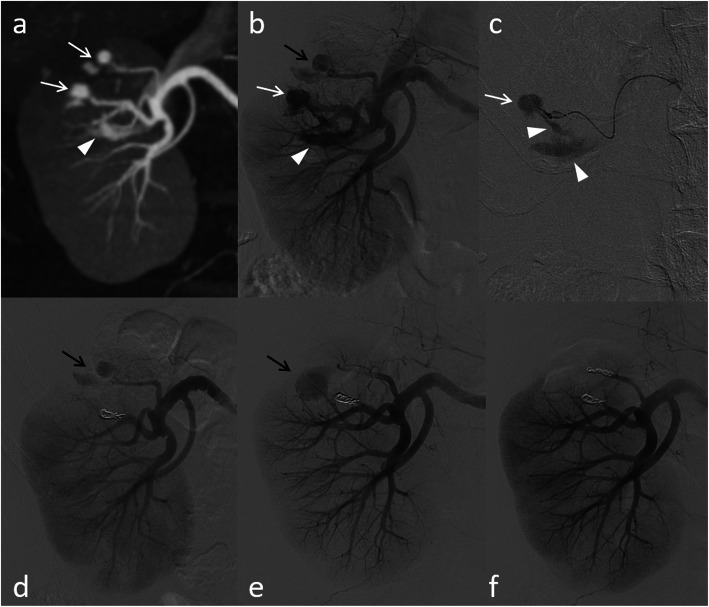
A case of undergoing transarterial embolization (TAE) twice in a 75-year-old man. a Two renal artery pseudoaneurysms (RAPs) (arrows) with arteriovenous fistula (AVF) (arrowhead) are observed on contrast-enhanced CT on postoperative day (POD) six. b, c, d Although one RAP (white arrows) with AVF (arrowheads) can be embolized using microcoils on POD eight, the other RAP (black arrows) cannot be embolized because of the difficulty of cannulation due to the acute angle of the branch. e, f The remaining RAP (black arrow), which is increased in size, is successfully embolized using microcoils at the second TAE after readmission with hematuria on POD 22
